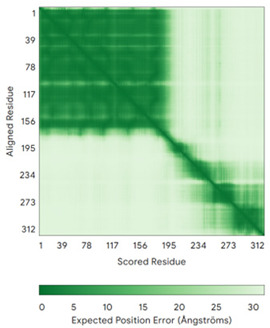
C9orf72 (Q96LT7)	- MSTLCPPPSPAVAKTEIALSGKSPLLAATFAYWDNILGPRVRHIWAPKTEQVLLSDGEITFLANHTLNGEILRNAESGAIDVKFFVLSEKGVIIVSLIFDGNWNGDRSTYGLSIILPQTELSFYLPLHRVCVDRLTHIIRKGRIWMHKERQENVQKIILEGTERMEDQGQSIIPMLTGEVIPVMELLSSMKSHSVPEEIDIADTVLNDDDIGDSCHEGFLLNAISSHLQTCGCSVVVGSSAEKVNKIVRTLCLFLTPAERKCSRLCEAESSFKYESGLFVQGLLKDSTGSFVLPFRQVMYAPYPTTHIDVDVNTVKQMPPCHEHIYNQRRYMRSELTAFWRATSEEDMAQDTIIYTDESFTPDLNIFQDVLHRDTLVKAFLDQVFQLKPGLSLRSTFLAQFLLVLHRKALTLIKYIEDDTQKGKKPFKSLRNLKIDLDLTAEGDLNIIMALAEKIKPGLHSFIFGRPFYTSVQERDVLMTF	Code: 6LT0 Technique: EM (Resol. 3.20 Å) Chain: C Residues: 1–481
